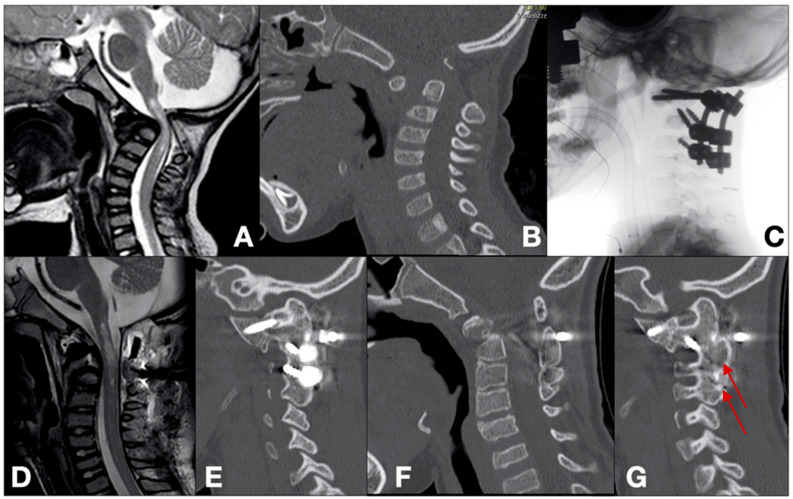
Pre-operative T2-weighted sagittal MRI scan (A). Pre-operative sagittal CT scan (B). Intra-operative radiographic acquisition (C). Post-operative T2-weighted sagittal MRI scan (D). 46-month follow-up sagittal CT scan demonstrating correct alignment, reduction in the malformation, and arthrodesis achievement (red arrows) (E–G).
